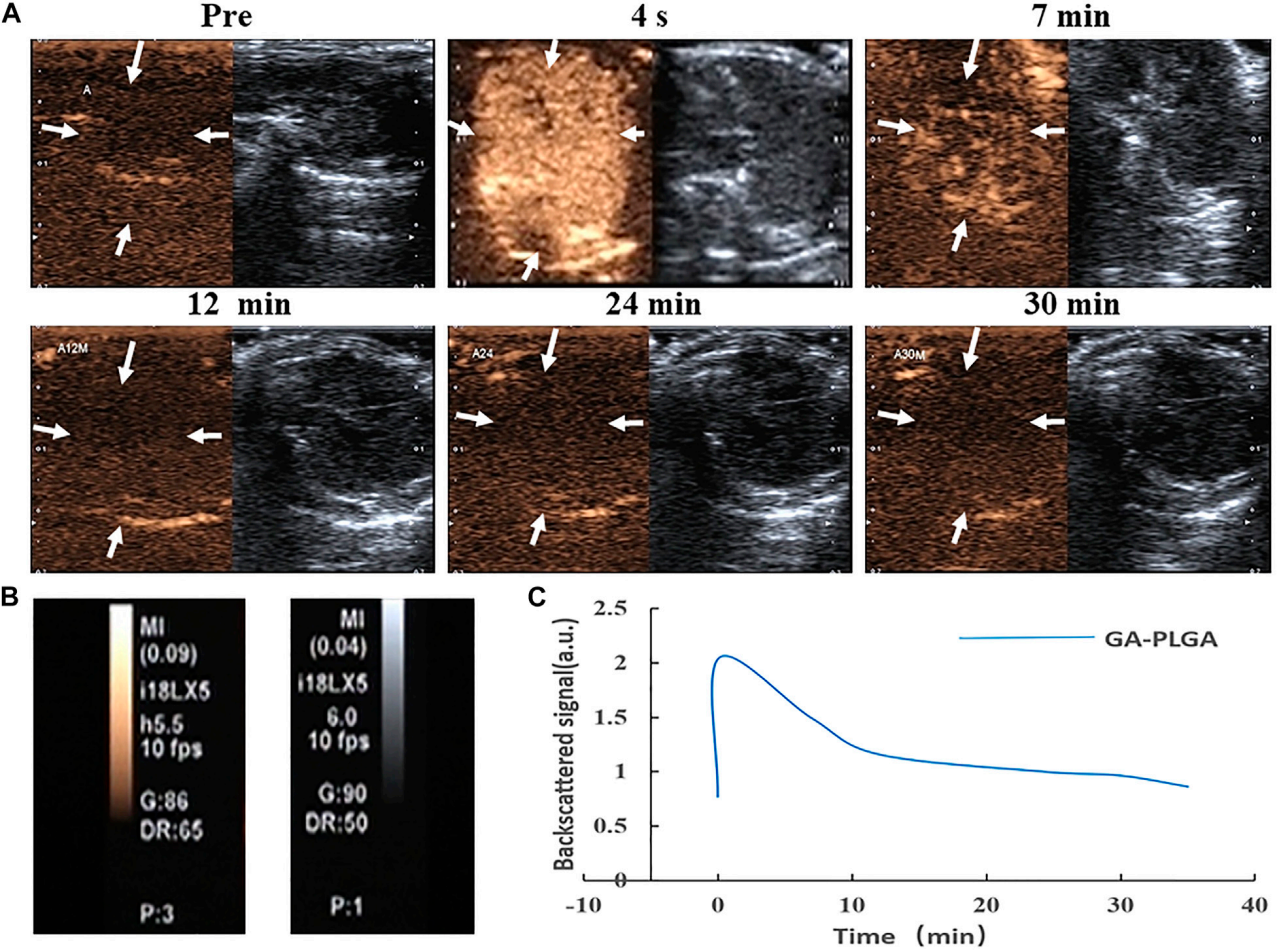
(A, B) Ultrasonic images of GA porous-lipid/PLGA MBs. (C) Changes in signal strength in the liver. After injection of GA porous-lipid/PLGA MBs, the liver imaging was rapidly enhanced, the signal gradually decreasing after 7 min and completely disappearing after 30 min.
